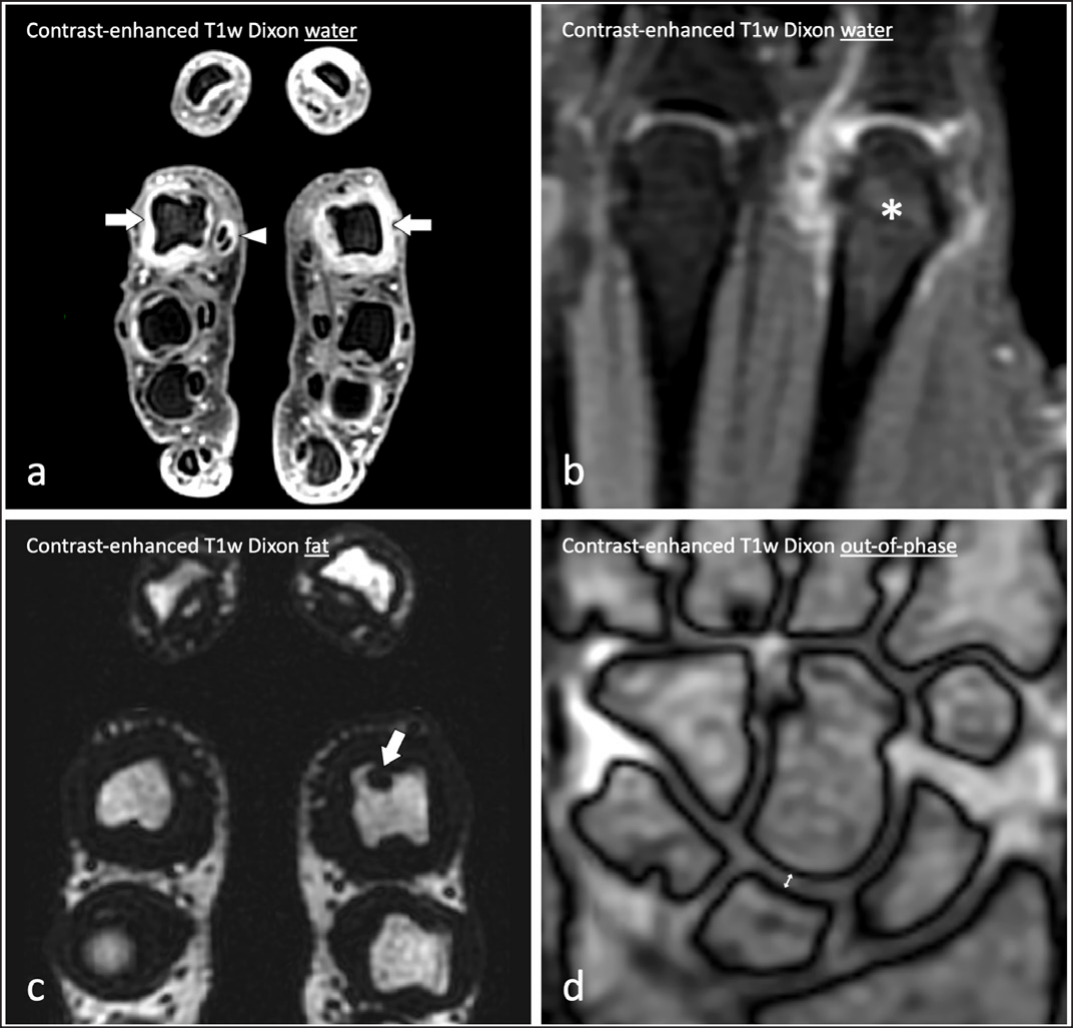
Contrast-enhanced T1-weighted Dixon MRI of the hands of a 28-year-old woman with early rheumatoid arthritis. (a) Contrast-enhanced T1-weighted Dixon water-only images in the axial plane demonstrate bilateral synovitis of the second metacarpophalangeal joints (arrows) and tenosynovitis of the flexor digitorum tendons of the right index finger (arrowhead). (b) Contrast-enhanced T1-weighted Dixon water-only images in the coronal plane also demonstrate osteitis of the head of the second right metacarpal bone (asterisk) while (c) contrast-enhanced T1-weighted Dixon fat-only images in the axial plane demonstrate a bone erosion of the head of the second left metacarpal bone (arrow). In addition, (d) contrast-enhanced T1-weighted Dixon out-of-phase images in the coronal plane allow to measure joint space width using India ink artifacts as landmarks and surrogates for the subchondral bone plates (up down arrow in the joint space between the lunate and capitate bones).
